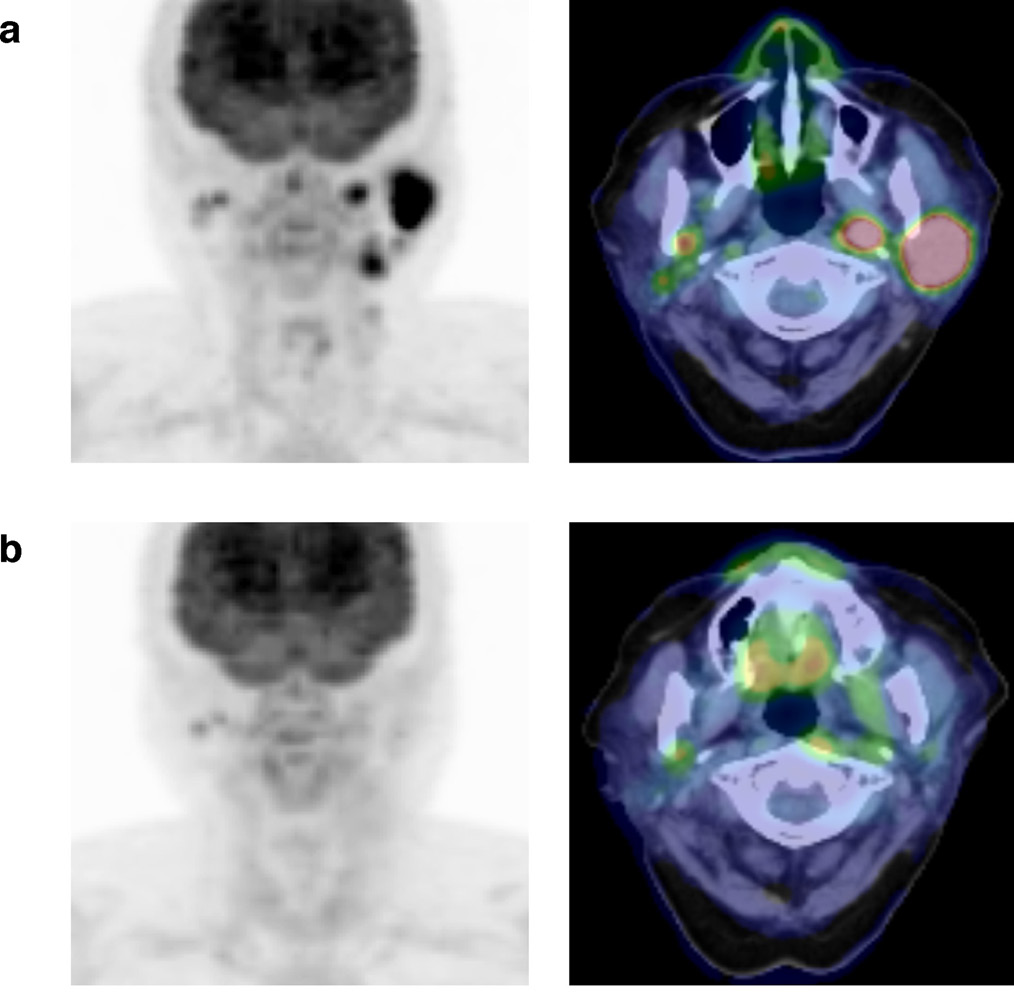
Images of pre-treatment ^18^F-FDG PET/CT (a), and images of post-treatment ^18^F-FDG PET/CT obtained at 4 months after the completion of the RTOG 8502 regimen (b). ^18^F-FDG PET, [^18^F]-fluoro-2-deoxy-D-glucose positron emission tomography
